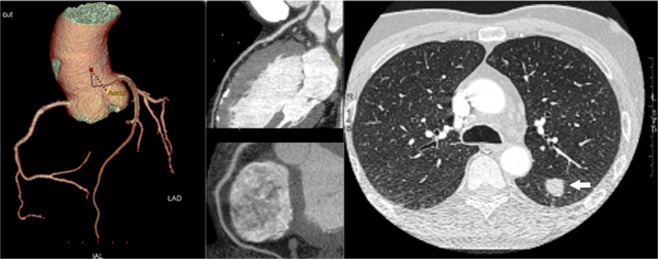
A case of coronary artery disease and lung cancer detection. CT images of the lungs showed a 20 mm pulmonary nodule (*white arrow*) in the left lobe (right panel). The patient underwent a complete diagnostic work-up, including positron emission tomography, and eventually underwent surgical resection. The cardiac CT revealed normal left coronary artery and right coronary artery (left panels).
